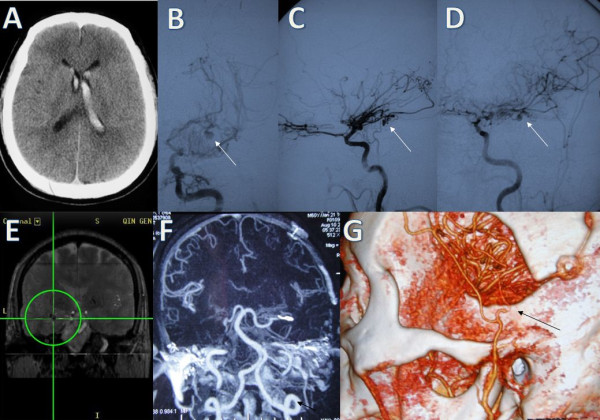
Case 1. (A) Computed tomography (CT) scan showing an intraventricular hemorrhage originating from the left subependymal area. (B), (C), (D) Left internal carotid angiograms revealing severe stenosis of the carotid fork accompanied with moyamoya vessels. There is an aneurysm (arrows) originating from the anterior choroidal artery (B frontal view, C lateral view, D oblique view). (E) Surgical planning with neuronavigation demonstrating the surgical trajectory to the aneurysm. (F), (G) CT scans 34 months after surgery showing the disappearance of the aneurysm and the patency of the bridge vessels (arrows).
